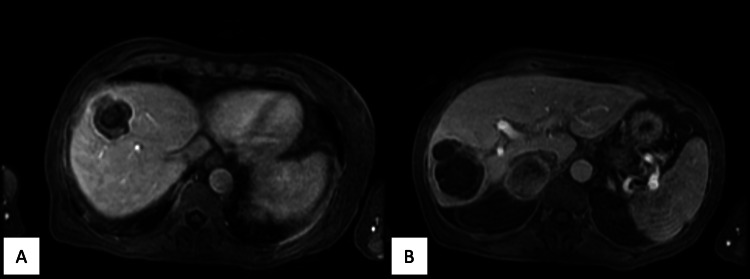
Axial T1 postcontrast late arterial phase MRI showing internal T1 hypointensity with rim and mild adjacent patchy parenchymal enhancement that persists through both phases of the superior (A) and inferior (B) lesions
